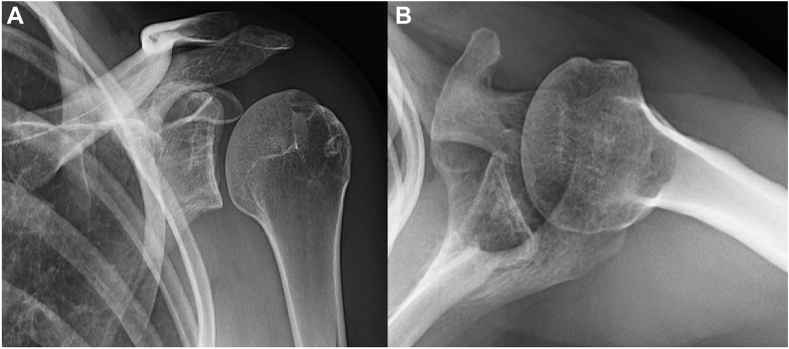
Grashey (A) and axial (B) radiographs of left shoulder at 31 months of follow-up. Both views demonstrate concentric reduction of the shoulder.
